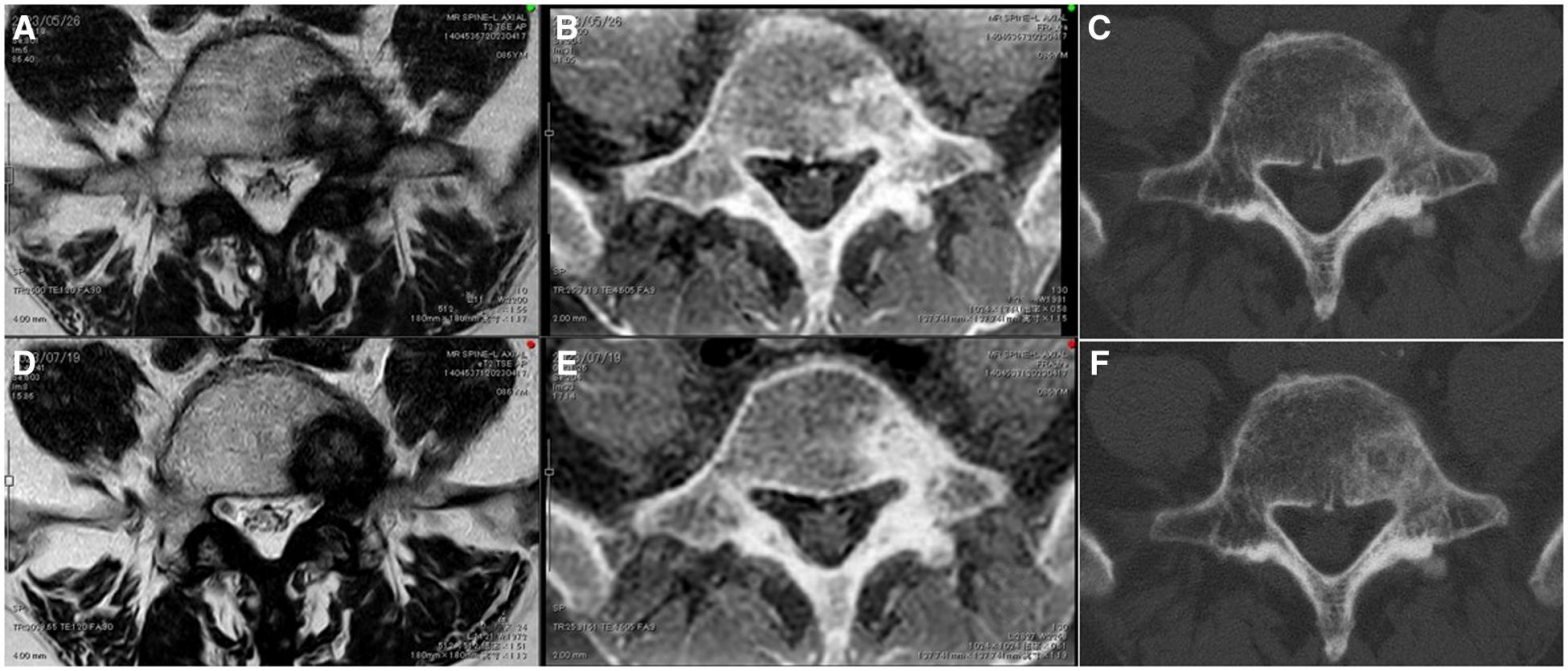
Comparison of images before and after radiation therapy. Panels A and D show T2-weighted images; panels B and E show CT-like magnetic resonance images, and panels C and F show plain CT images. Panels A, B, and C show images before radiotherapy for bone metastasis, whereas panels D, E, and F show images after radiotherapy. Panels B and E show an increase in the area of bone sclerosis before and after radiotherapy.
